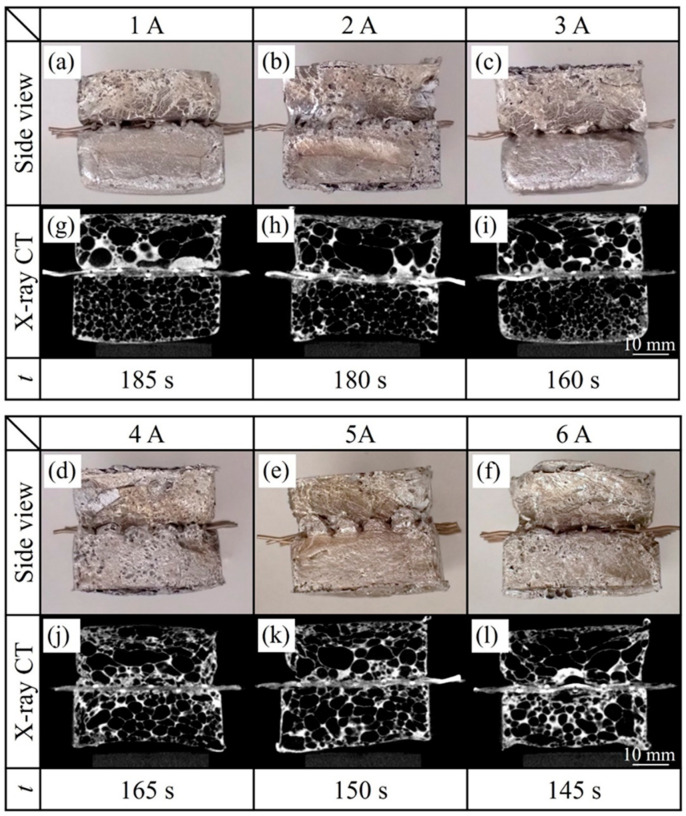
Side views of the resulting two-layer aluminum foam, an X-ray CT image of a longitudinal section near the center of the sample, and the time *t* from the start of heating the precursor to the end of the heating process for heating and foaming two precursors with a gap between them. “Halogen lamps II” on the underside of the ADC12 precursor were varied from 1 A to 6 A.
